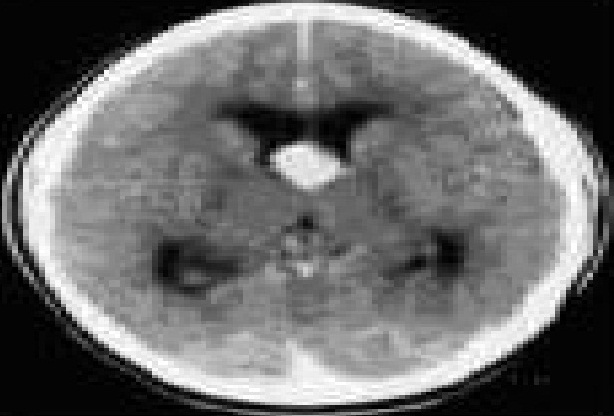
CT scan with contrast administration
